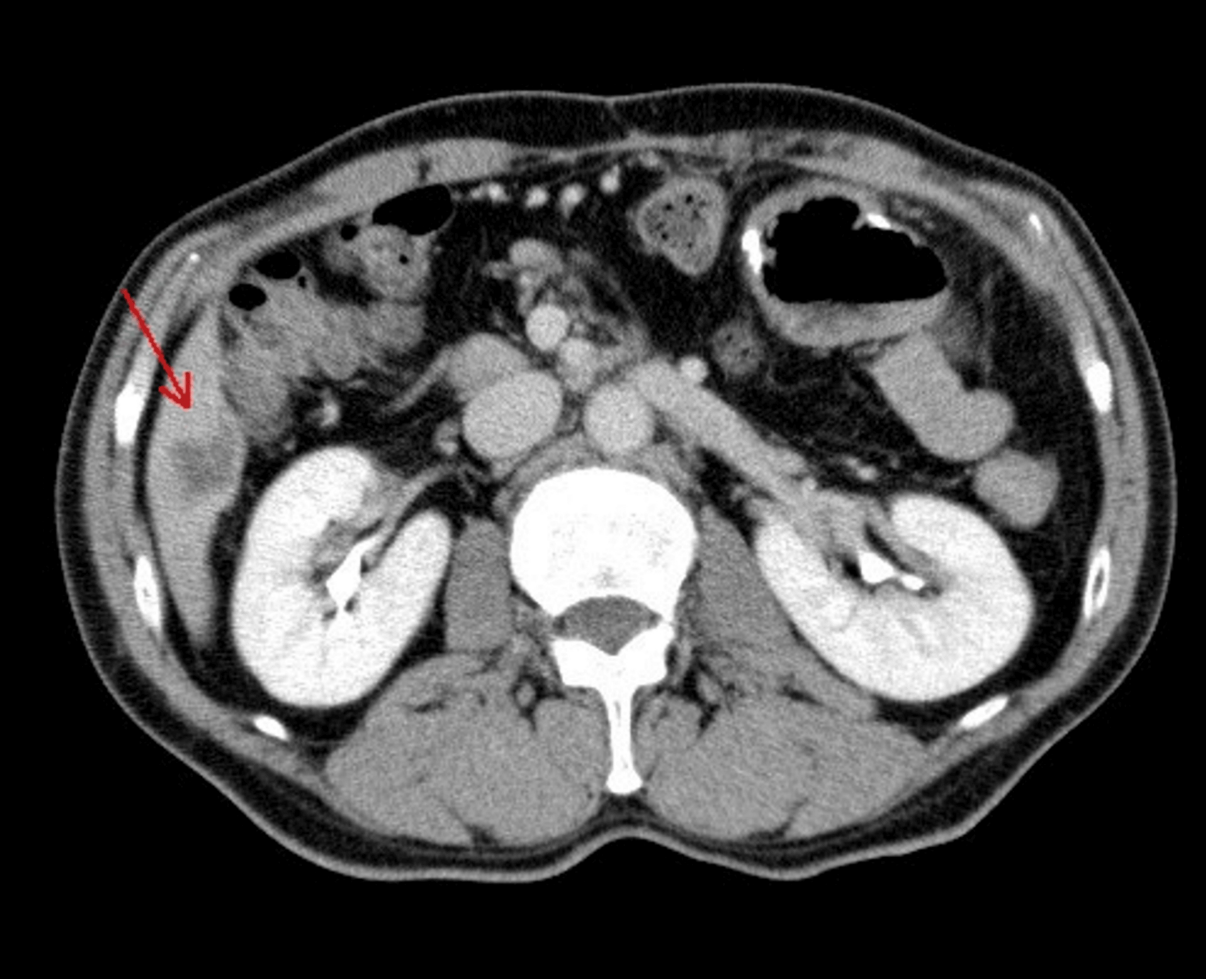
Contrast‑enhanced computed tomography prior to chemotherapy (February 2024) Contrast-enhanced computed tomography performed in February 2024, showing newly developed metastatic lesions in the liver (arrows) after pancreaticoduodenectomy.
